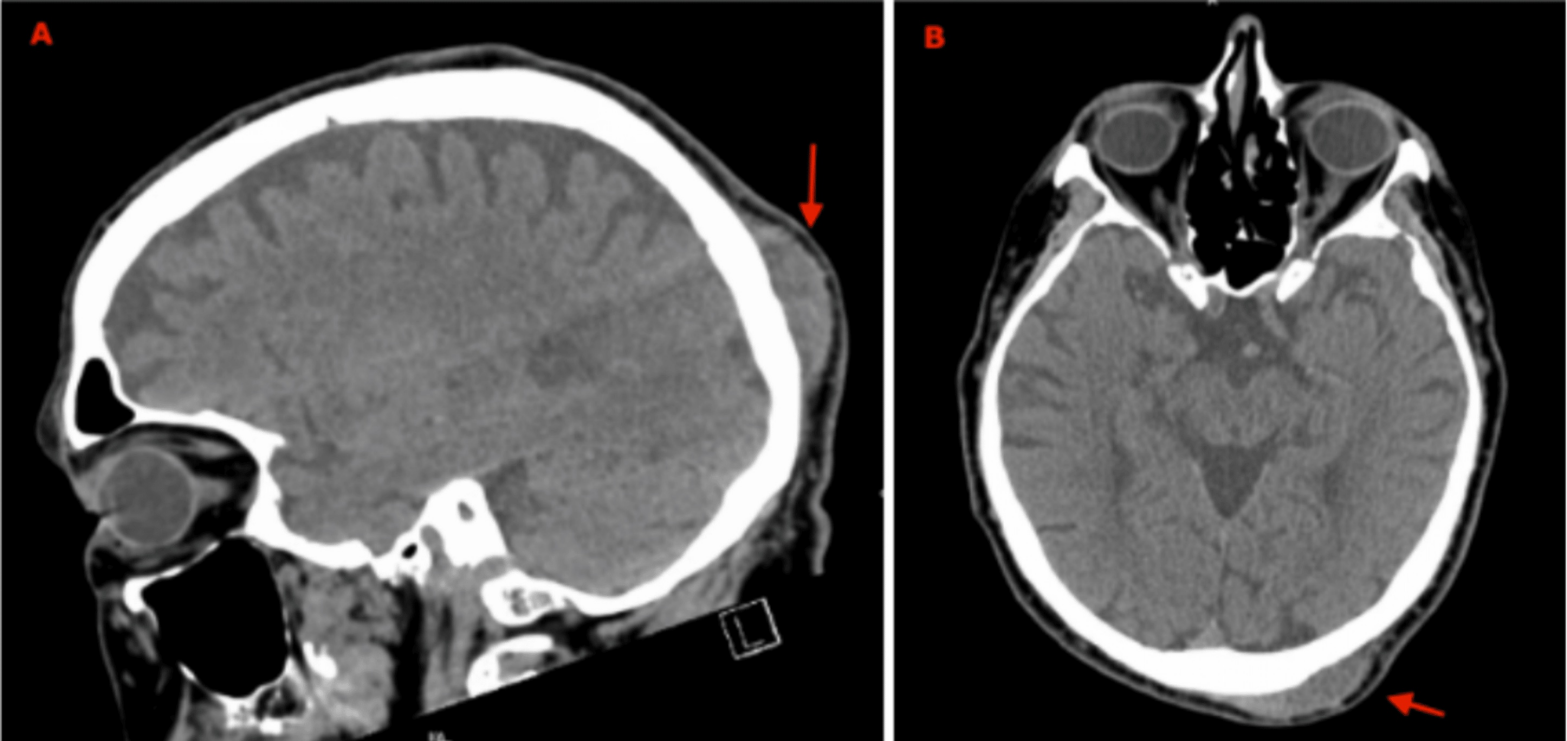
CT head showing 5 cm subcutaneous posterior left scalp mass abuts the calvarium with no underlying bony abnormality, periosteal reaction, or measles effect on the underlying brain parenchyma. A: Sagittal view of the head with the lesion (red arrow). B: Transverse view of the head with the mass (red arrow).
